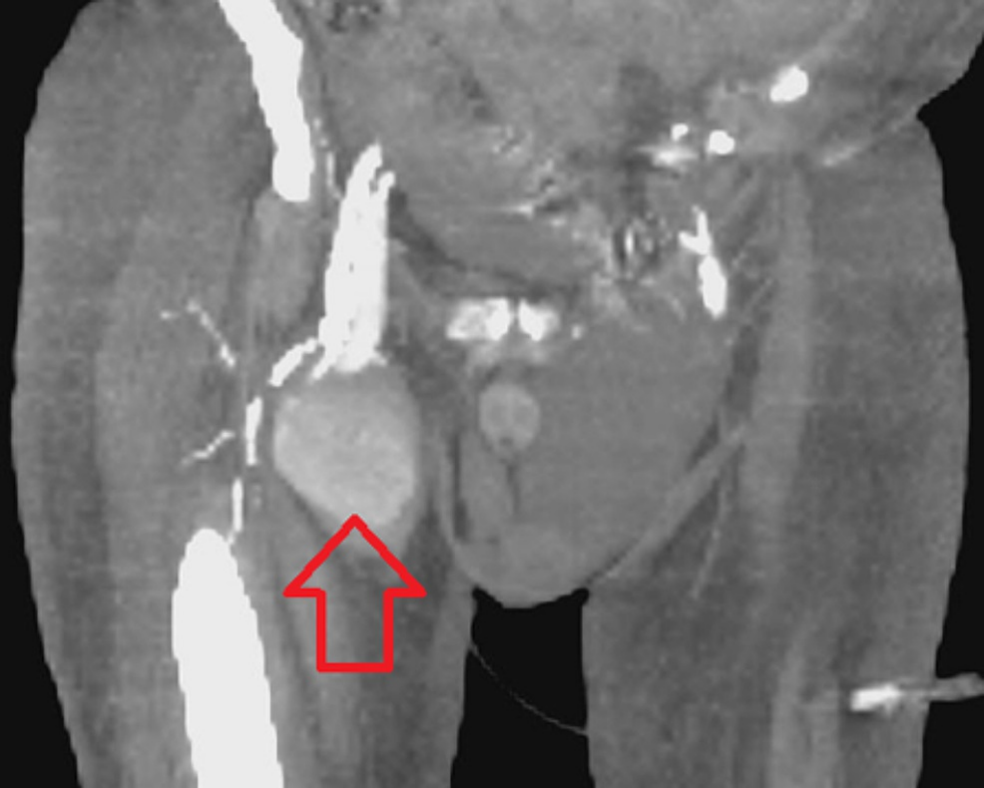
CT angiography of right lower extremity. Red arrow showing large right superficial femoral artery pseudoaneurysm.
